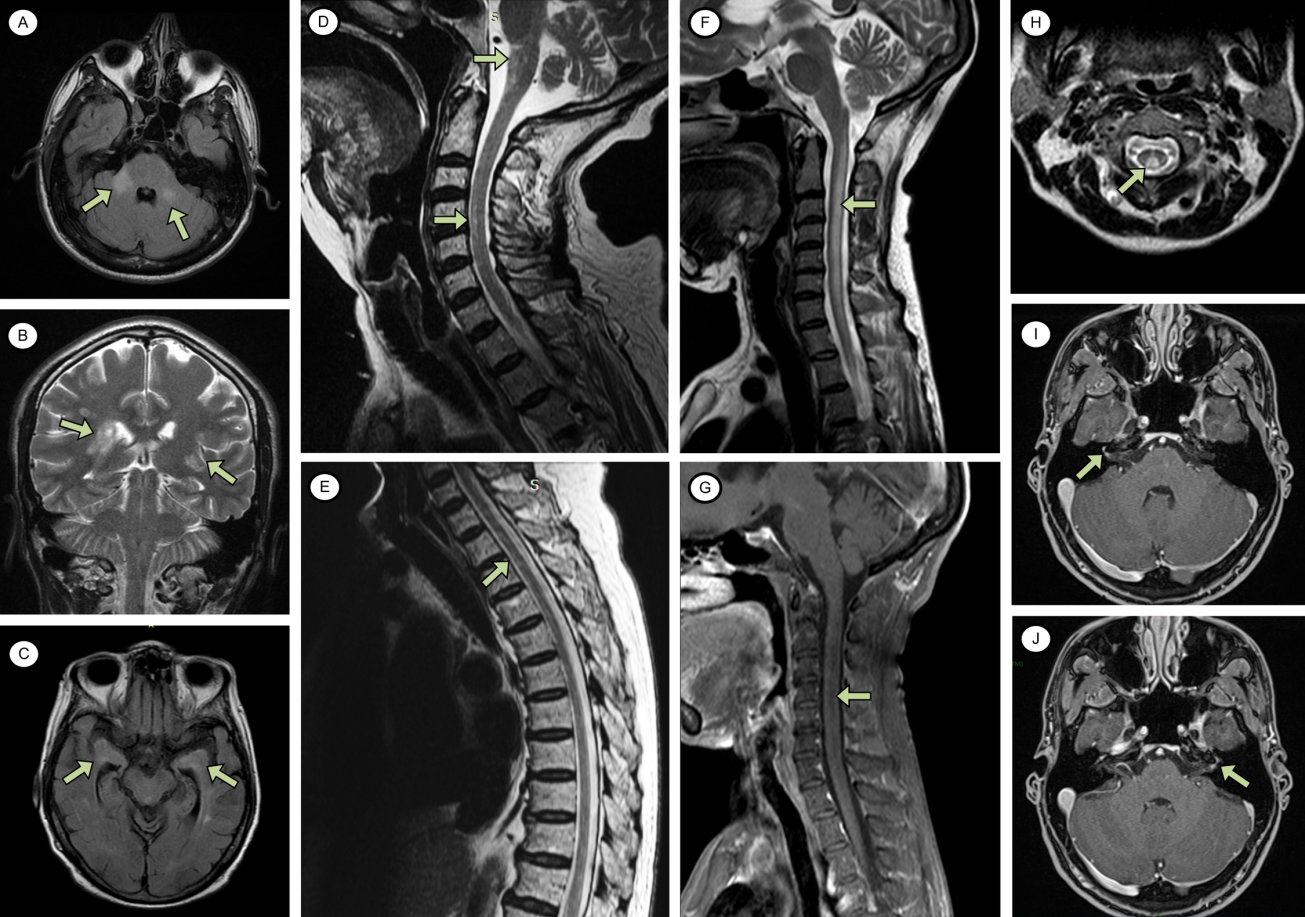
Central nervous system (CNS) imaging abnormalities in patients with evidence of Zika, chikungunya and/or dengue virus infection. A: Encephalomyelitis in a patient with CNS Zika and systemic dengue infection (patient 3). Fluid attenuation inversion recovery [FLAIR] signal abnormality involving the middle cerebellar peduncles, more marked on the right (axial scan). B: Acute disseminated encephalomyelitis in a patient with systemic chikungunya infection (patient 20). Confluent areas of T2 signal abnormality suggesting neuroinflammation consistent with demyelination (coronal scan). C, D, E: Encephalomyelitis with subclinical meningitis in a patient with CNS Zika + chikungunya infection (patient 9). FLAIR signal abnormality involving the medial temporal lobes, amygdala, and a small area of abnormality adjacent to the temporal horn of the left lateral ventricle (C, axial scan). High signal intensity on T2-weighted images in the anterior medulla, and anterior cervical and thoracic cord (D and E, sagittal scans). F, G, H: Myelitis in a patient with CNS Zika + dengue and systemic chikungunya infection (patient 2). Extensive intramedullary signal abnormality of the cervical cord, without evidence of contrast enhancement (F, sagittal T2-weighted scan; G, sagittal T1-weighted scan with gadolinium; H, axial T2-weighted scan). I, J: Facial diplegia with paraesthesia in patient with CNS Zika + chikungunya infection (patient 4). Bilateral facial nerve enhancement on T2-weighted images with gadolinium (axial scan).
